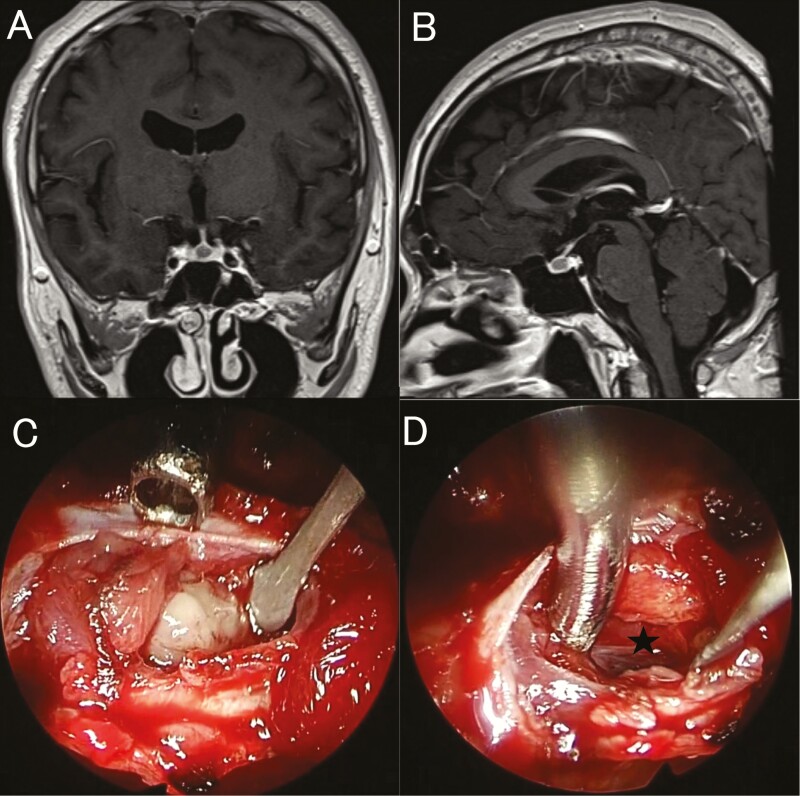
Magnetic resonance imaging (MRI). Preoperative contrast-enhanced coronal (A) and sagittal (B) MRIs showing a 5 × 6 × 8 mm less-enhanced mass lesion inside the pituitary gland. Intraoperative images show a well-circumscribed whitish tumor after splitting the pituitary gland (C), which was completely removed via a fine dissection plane. D: The arachnoid membrane can be seen over the resection cavity (star).
